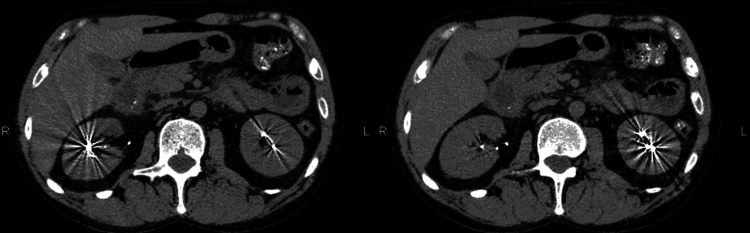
Non-enhanced CT (NECT) scans in the axial plane (a and b) depict a person who has been poisoned by heavy metals, exhibiting characteristic metallic artifacts on both sides. Heavy metals such as lead, mercury, arsenic, and cadmium can accumulate within the body tissues, including the bones and organs, leading to toxic effects. The metallic artifacts produce characteristic radiopaque shadows. This figure is a republished image, permission was obtained from the original publisher, https://epos.myesr.org/poster/esr/ecr2018/C-2802
